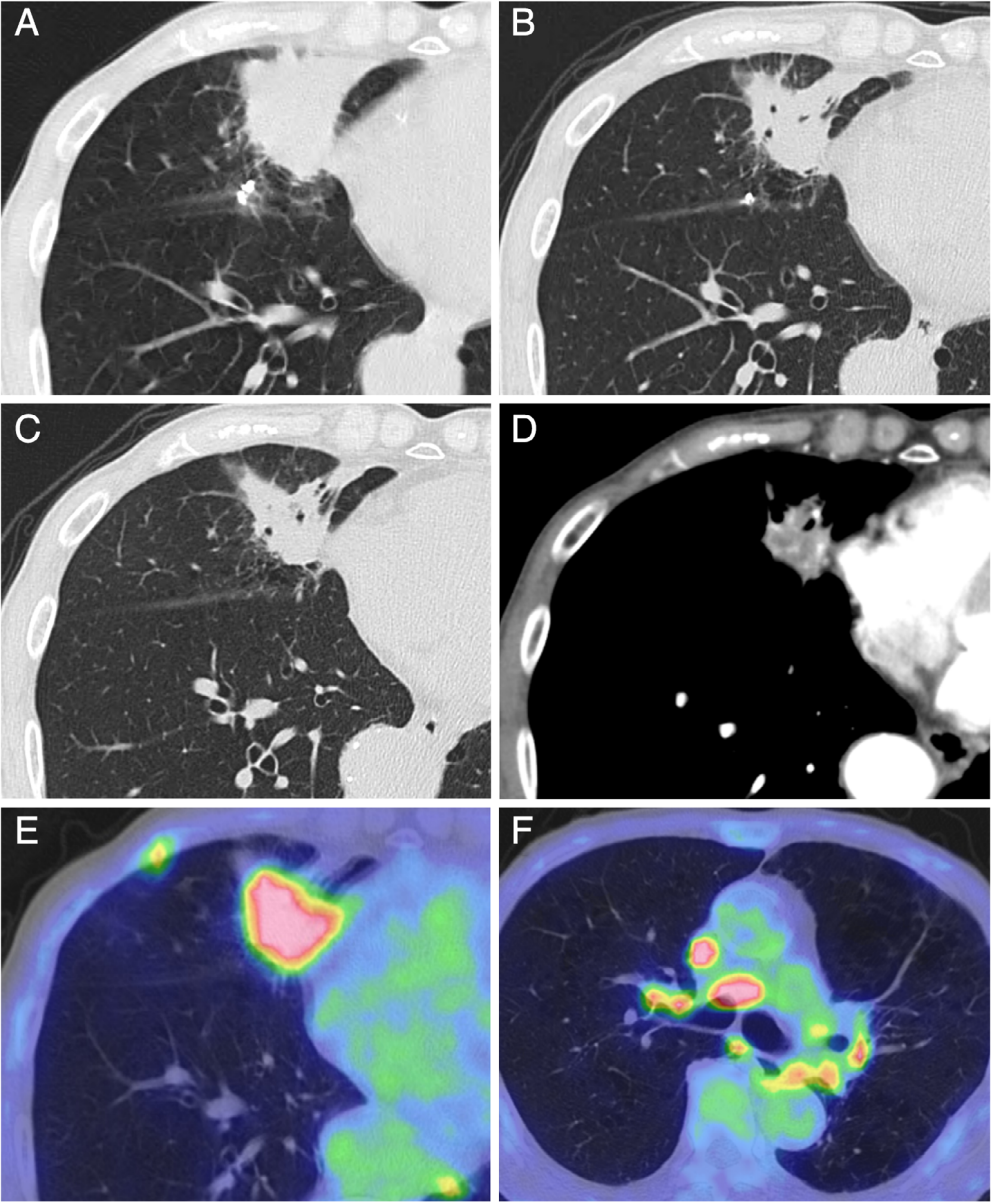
(A) Chest computed tomography (CT) scanning reveals a mass in the right middle lobe. (B) Tosufloxacin was administered for pneumonia, which shows reduction of the mass after one month. (C) CT scanning performed again after an additional one month unexpectedly shows the persistence of the tumour shadow. (D) An enhanced chest CT scan (mediastinal window setting). (E) Positron emission tomography depicts fluorodeoxyglucose uptake in the mass. (F) Fluorodeoxyglucose uptake is also observed in the lymph nodes.
